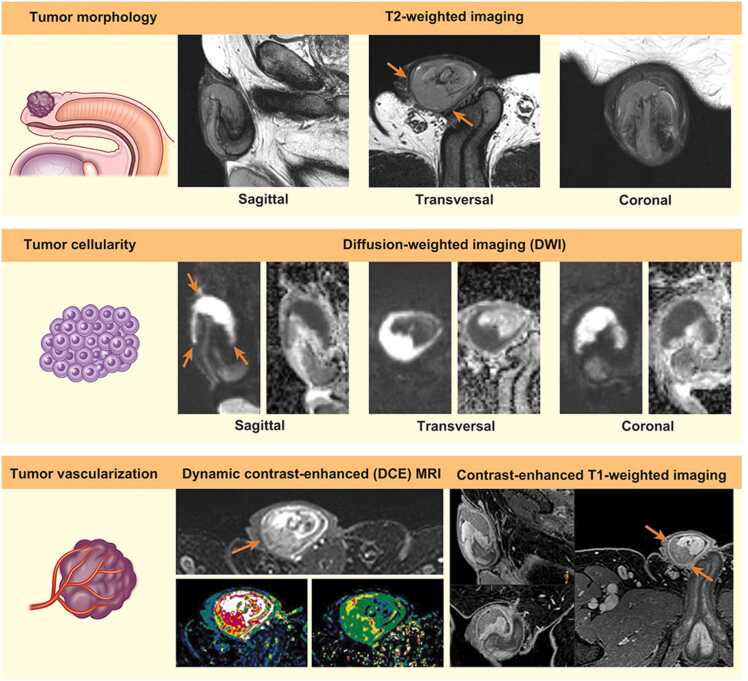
Principles of the multi-parametric magnetic resonance imaging protocol for preoperative evaluation of primary penile carcinoma. The interpretation is based on the integrated assessment of tumor morphology (upper row), cellularity (middle row), and vascularization (lower row).
